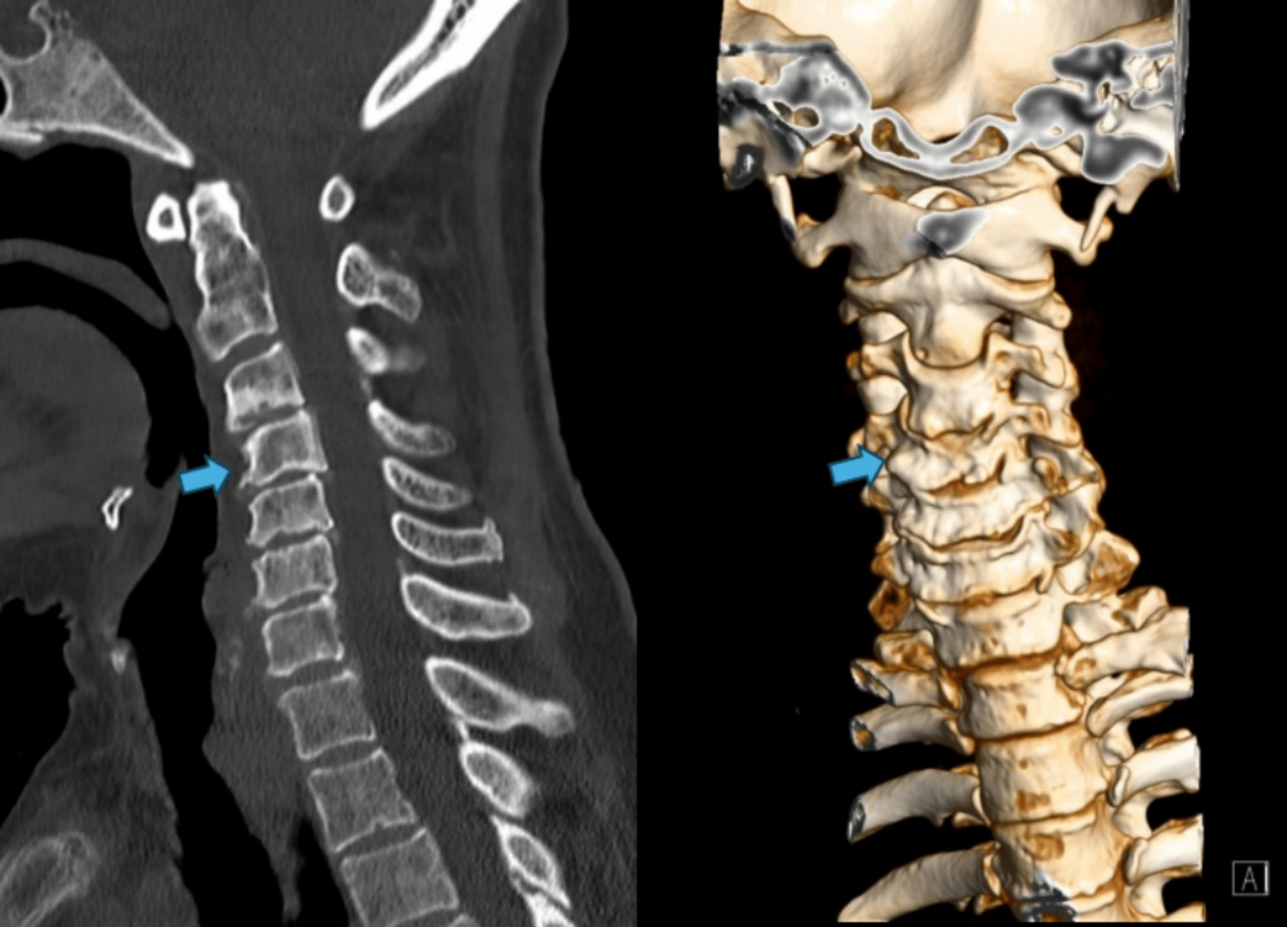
Sagittal and coronal plane on CT imaging (June 2022) There are no traces of recent fractures in the discovered planes, nor images suggestive of intracanal hematomas. The spinal canal has specific dimensions, but is narrow due to degenerative changes, particularly in the segment between C3 and C6. Kyphotic inversion of the cervical curvature, with incipient retrolisthesis of C4, and slight scoliotic deviation of right convexity, centered on C3-C4, in the study position. The spinal canal has specific dimensions, although it is narrow due to degenerative changes, particularly in the segment between C3 and C6 (arrows), highlighting discopathies with reduced interbody height and sclerosis of the vertebral platforms, uncarthroses, disc protrusions/disc-osteophytes, mild interapophyseal arthrosis, and calcifications of the yellow ligaments.
